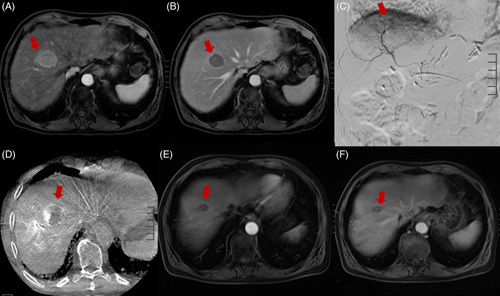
Imaging characteristics of early-stage HCC before, during, and after radiation segmentectomy. (A and B) Preradiation segmentectomy MRI images of a solitary, arterially enhancing hepatic segment 8 mass (A) demonstrating washout on portal venous phase (B) compatible with LI-RADS 5 HCC lesion (red arrow). (C and D) Angiographic digital subtraction image of segment 8 lesion at the time of radiation segmentectomy (C) with correlative, intraprocedural cone beam CT image (D) demonstrating lesion of interest (red arrow). (E and F) Approximately 6 months postradiation segmentectomy MRI images. Segment 8 lesion demonstrates no residual arterial enhancement with a slight decrease in size on portal venous phase (E) compatible with treated HCC (red arrow) (F). Abbreviation: LI-RADS, Liver Imaging Reporting and Data System
